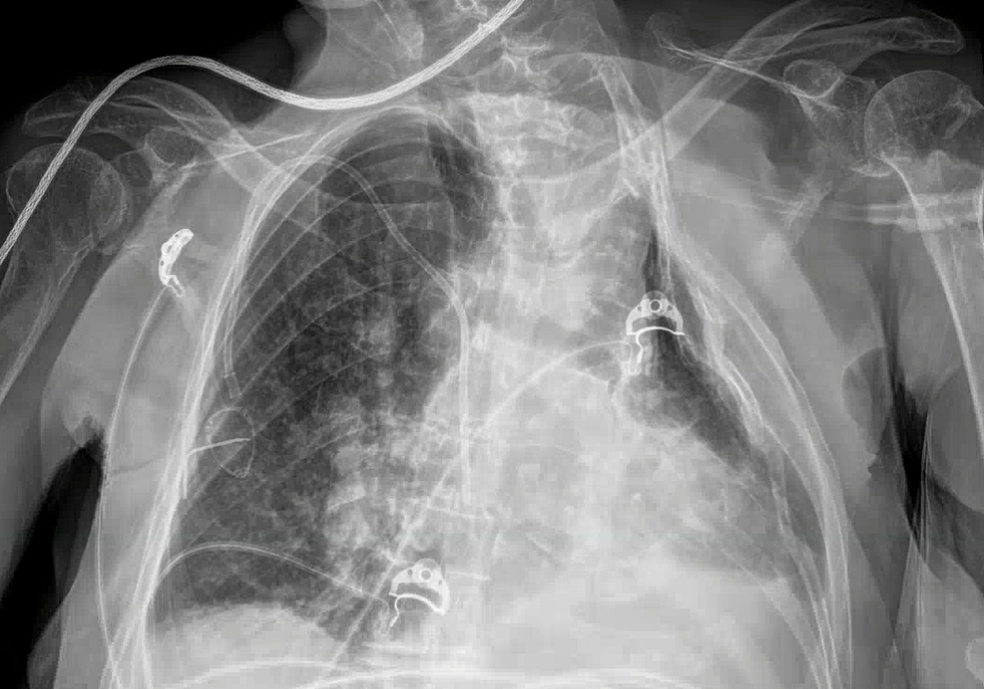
Chest X-Ray After Thoracentesis
